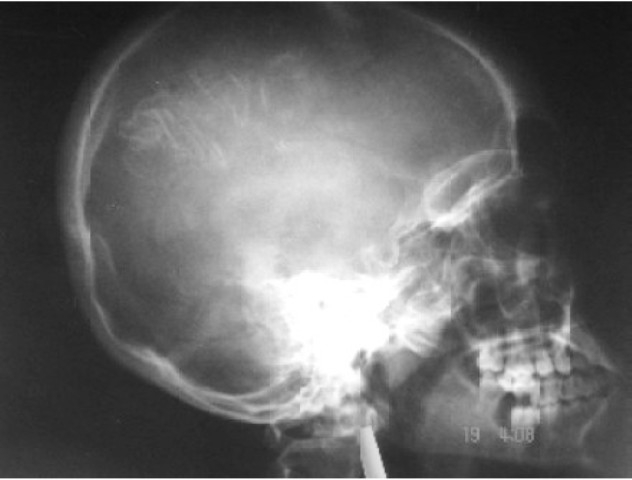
Lateral skull x-ray showing Tram Track calcifications in the left frontoparietal region
